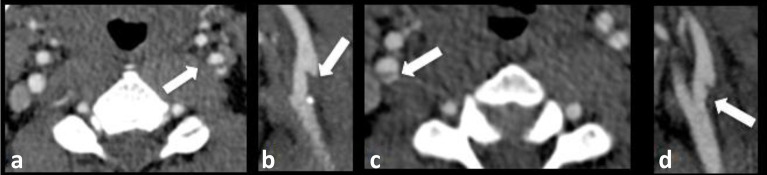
Bilateral carotid webs. CTA images of a 39-year-old male patient who presented with an acute left M1 occlusion with a background history of a prior left MCA infarct. (a and b) Axial and sagittal CTA images demonstrate a carotid web arising from the posterior wall of the proximal left ICA. (c and d) Axial and sagittal CTA images demonstrate a carotid web arising from the posterior wall of the proximal right ICA. CTA, CT angiogram; ICA, internal carotid artery; MCA, middle cerebral artery.
